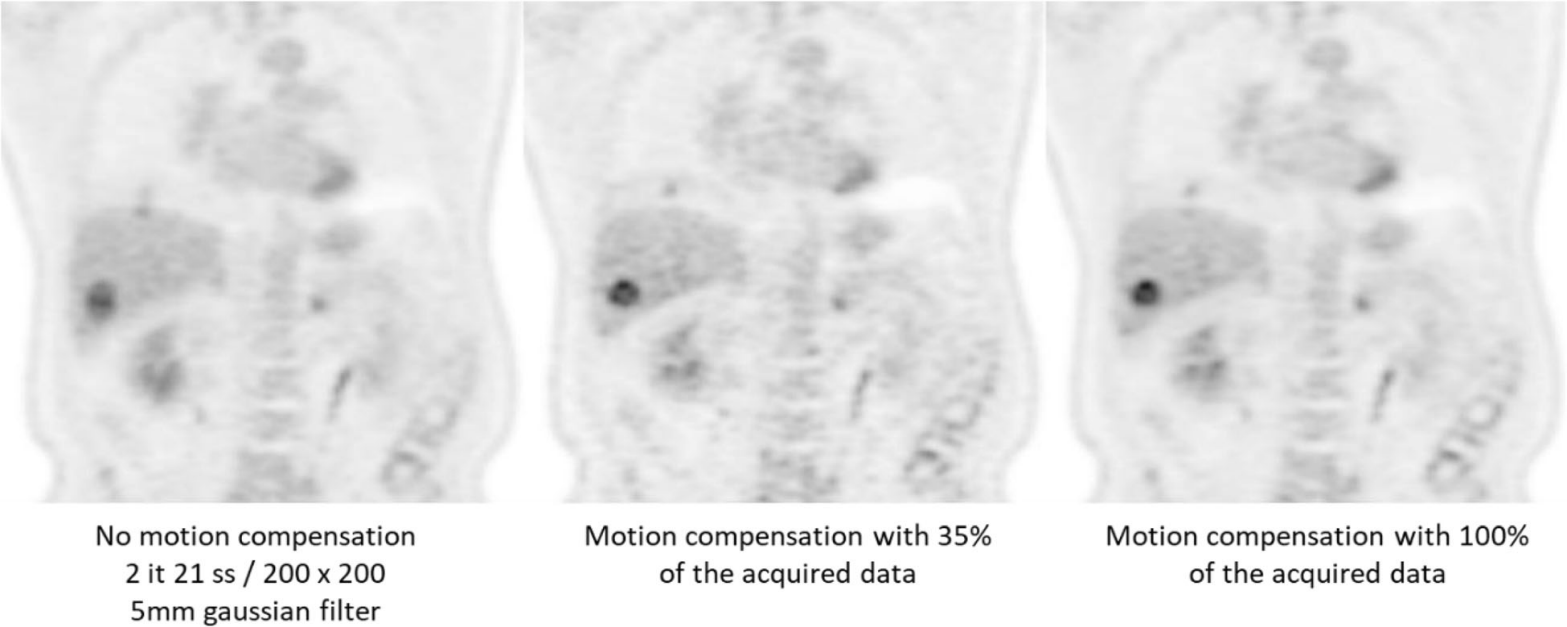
[18F]-FDG PET images of a 60-y/o male (362 MBq, BMI = 28) with colorectal cancer. Images show lewsions in the liver and right lower lung that are affected by motion-induced blurring. Left: without motion compensation, middle: with amplitude motion compensation that utilizes 35% of the acquired data resulting in noisy images but reduced motion artifact, and right: with amplitude motion compensation that utilizes all the acquired data resulting in lower image noise and motion artifact
